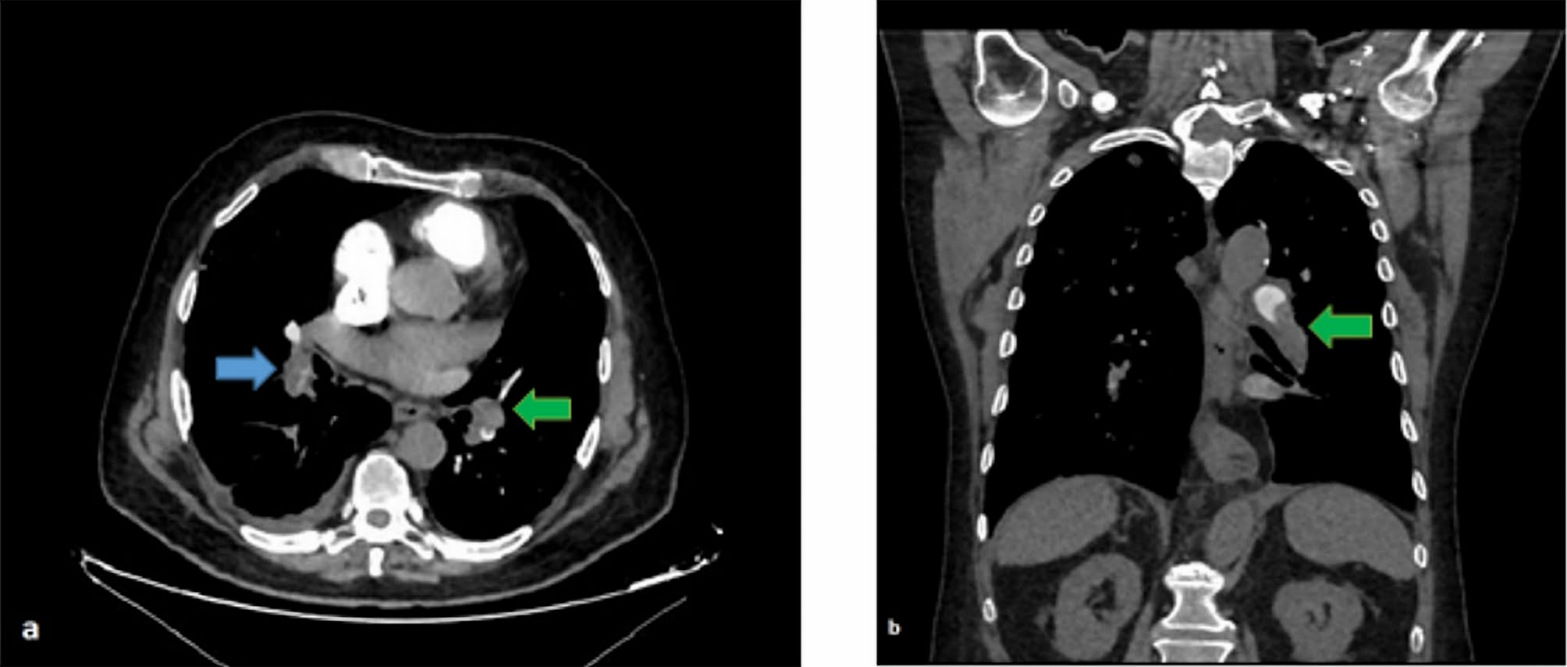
Computed tomography pulmonary angiography images of a 72-year-old male patient. A thromboembolic filling defect is observed to cause 50–99% stenosis in the lower lobar branches of both pulmonary arteries in the axial image(a, blue and green arrows).Coronal section view of the thrombus in the proximal left lower lobar branch(b, arrow)
